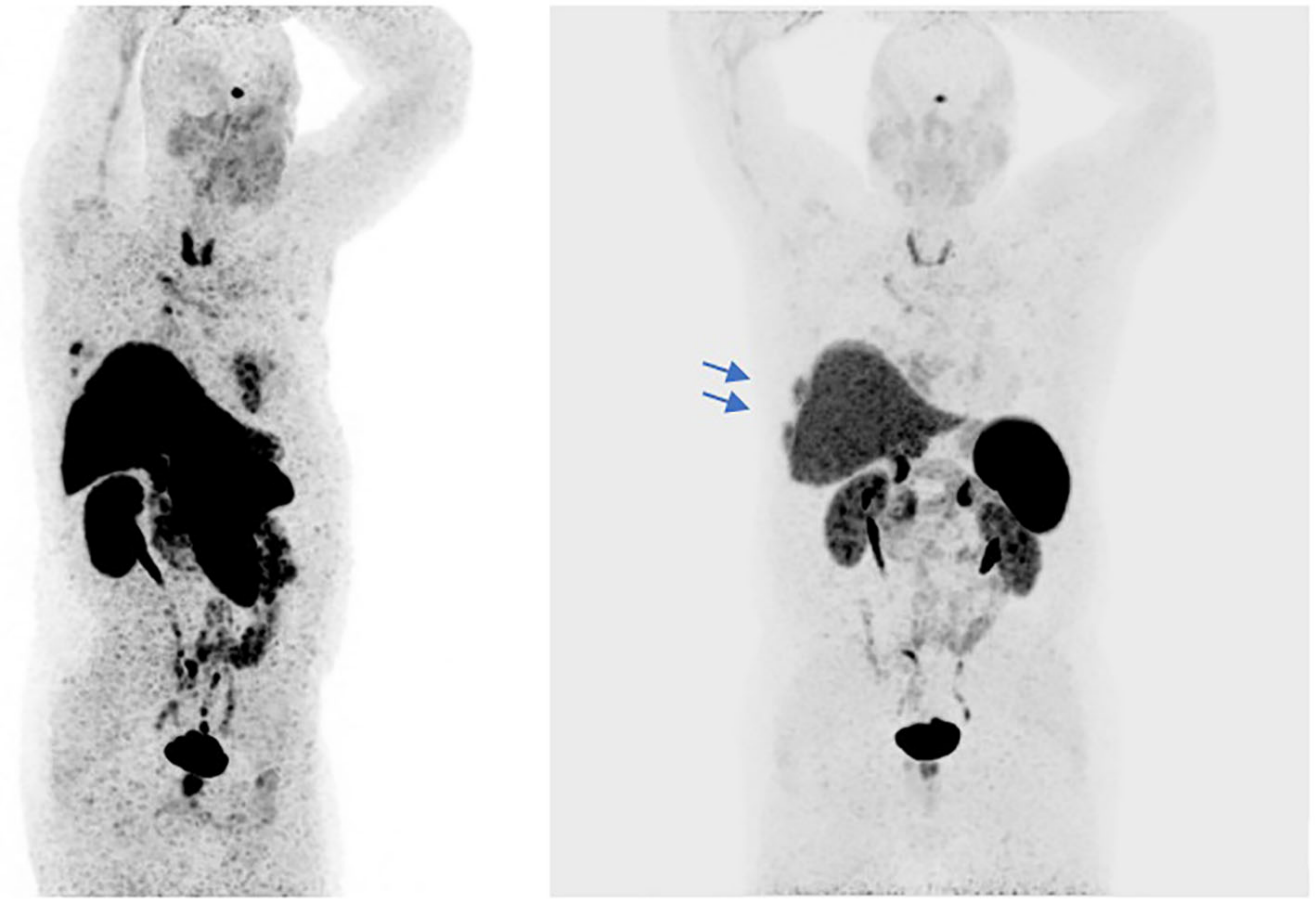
The ^68^Ga-PET/CT shows areas of slight somatostatin receptor uptake in the right subpleural paracardiac area, standardized uptake value (SUVmax = 5.2), and some pre-vascular (SUVmax = 2.3), retrosternal (SUVmax = 3.2), Barety lodge (SUVmax = 3), and right peribronchial (SUVmax = 5.4) node uptake (red arrows). Some areas in the dorsal segment of the upper lobe of the right lung and in middle lobe of the right lung showed no uptake. Slight uptake of the radiopharmaceutical product in the sternal body (SUVmax = 5.9) and the VI and VII right ribs (SUVmax = 8.6) (blue arrows).
